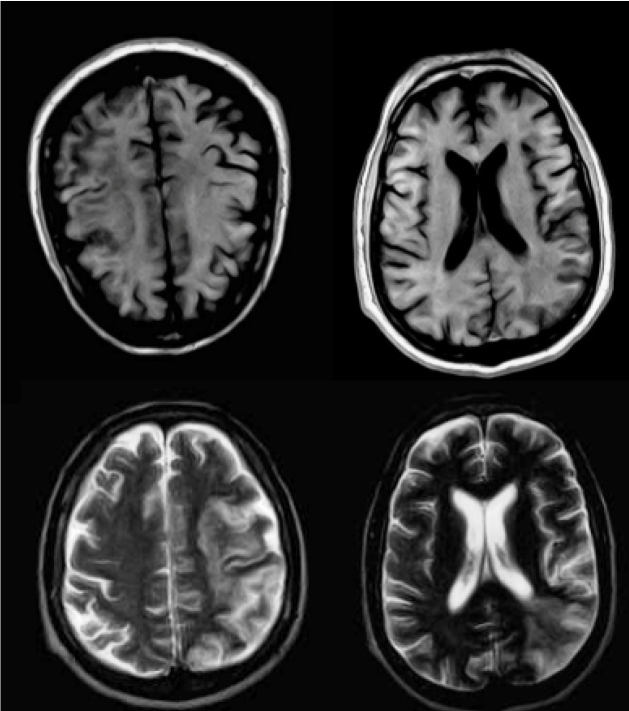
Magnetic resonance imaging of the skull in T1 showing hyposignal in the territory of the artery (left cerebral artery), with high intrinsic cortical signal, and hyperintensity in the T2 sequence.
